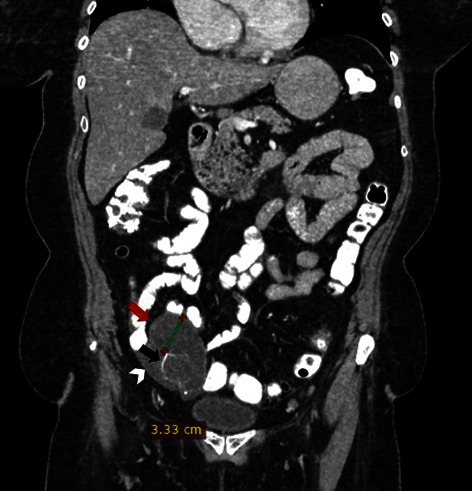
Coronal CT: located in the anatomic region of the right iliac fossa, a low-attenuation cystic lesion that measures 3.3 cm × 6.5 cm with asymmetric wall thickening (red arrow) and focal calcification (black arrow). Peritumoral ascitic fluid can be detected (white arrow).
